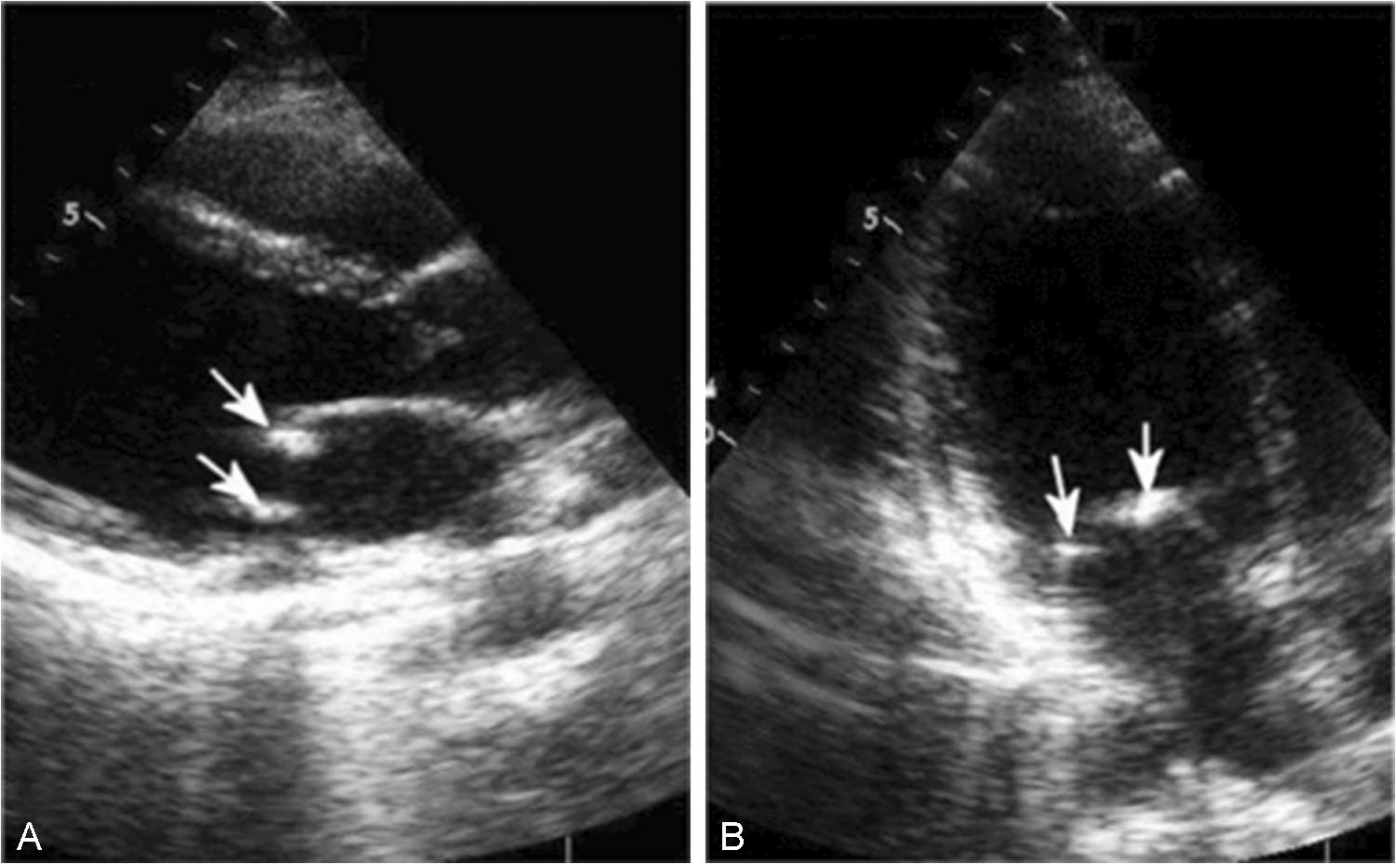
A young patient with antiphospholipid syndrome and ischemic stroke at the age of 21 years. Mitral leaflets are thickened with round nodules at the edges seen in the parasternal (A) and apical (B) echocardiographic window. Nodules may serve as the base for clot formation and increase the risk of embolization
